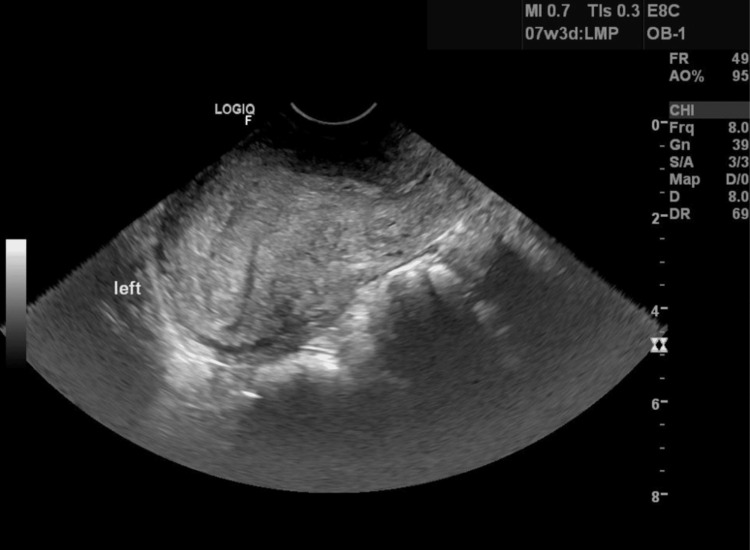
A transvaginal ultrasound of the intrauterine cavity at the time of diagnosis of the pregnancy within the rudimentary uterine horn.
